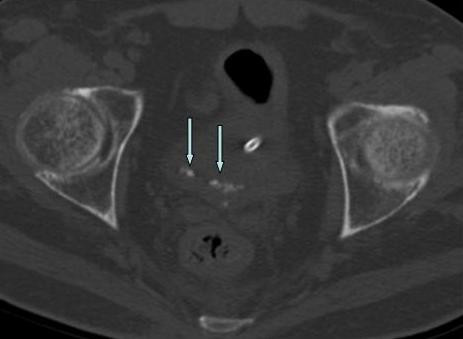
Axial pelvic CT scan without contrast. Note the multiple calcifications located in the dependent portion of the bladder demonstrating interval change in appearance (arrows).
